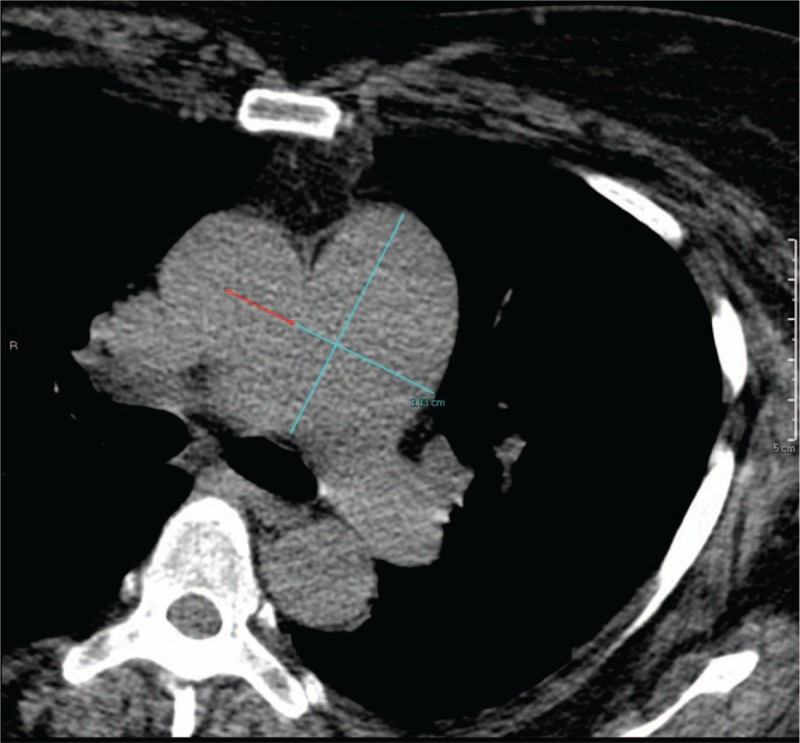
Measurement of main pulmonary artery diameter (mPAD). Axial gated-computed tomography (CT) image demonstrating measurement method for determining mPAD. The mPAD is measured along the line that originates from the center of the ascending thoracic aorta and passes perpendicular to the long axis of the main pulmonary artery, at the level of the pulmonary artery bifurcation.
